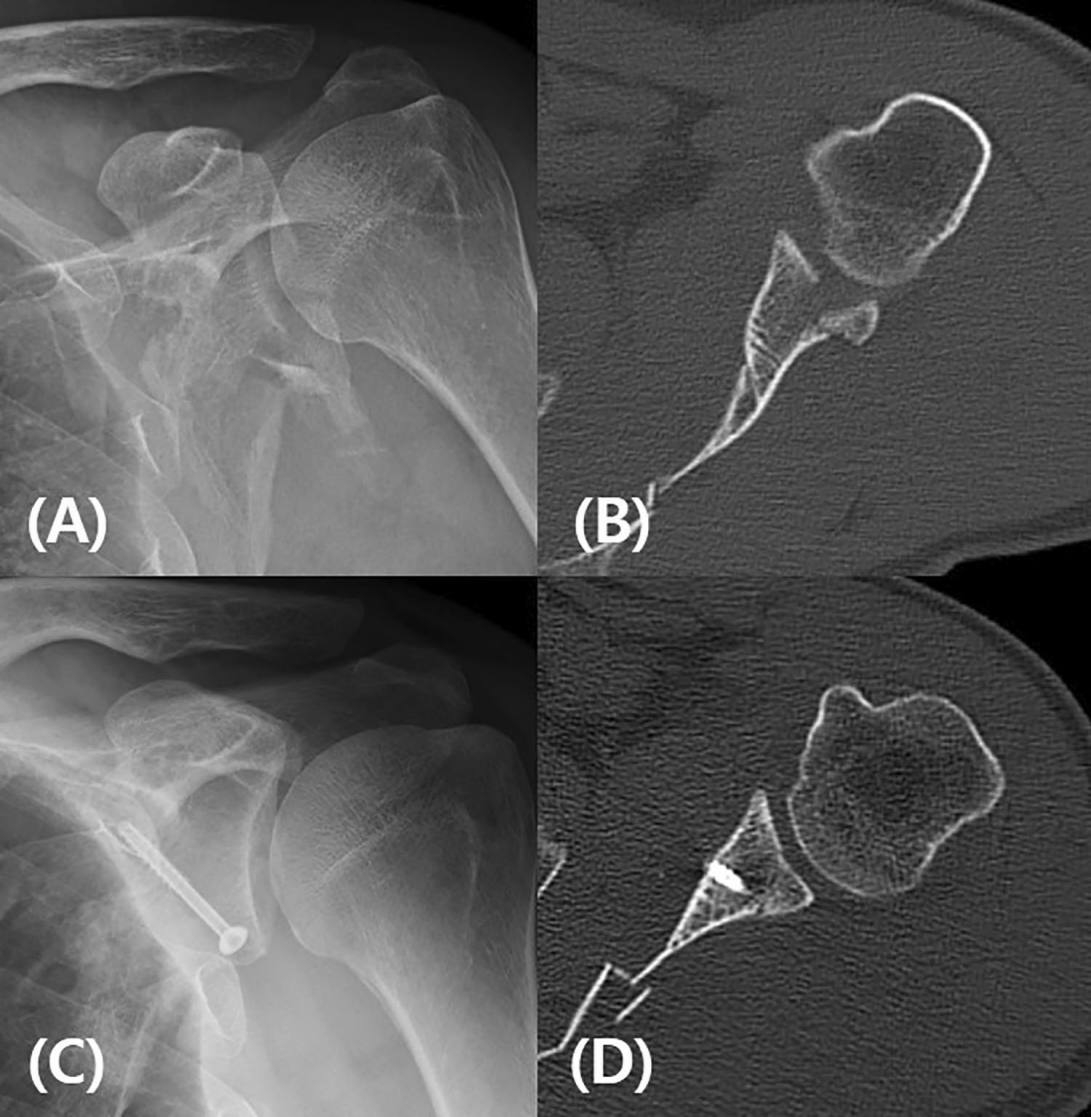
(A) Preoperative simple radiography and (B) three-dimensional computed tomography (3D CT) show a displaced postero-inferior glenoid fossa fracture. (C) A 4.0-mm partial-thread cannulated screws are inserted, and (D) postoperative CT shows excellent congruency of the articular surface of glenoid fossa.
